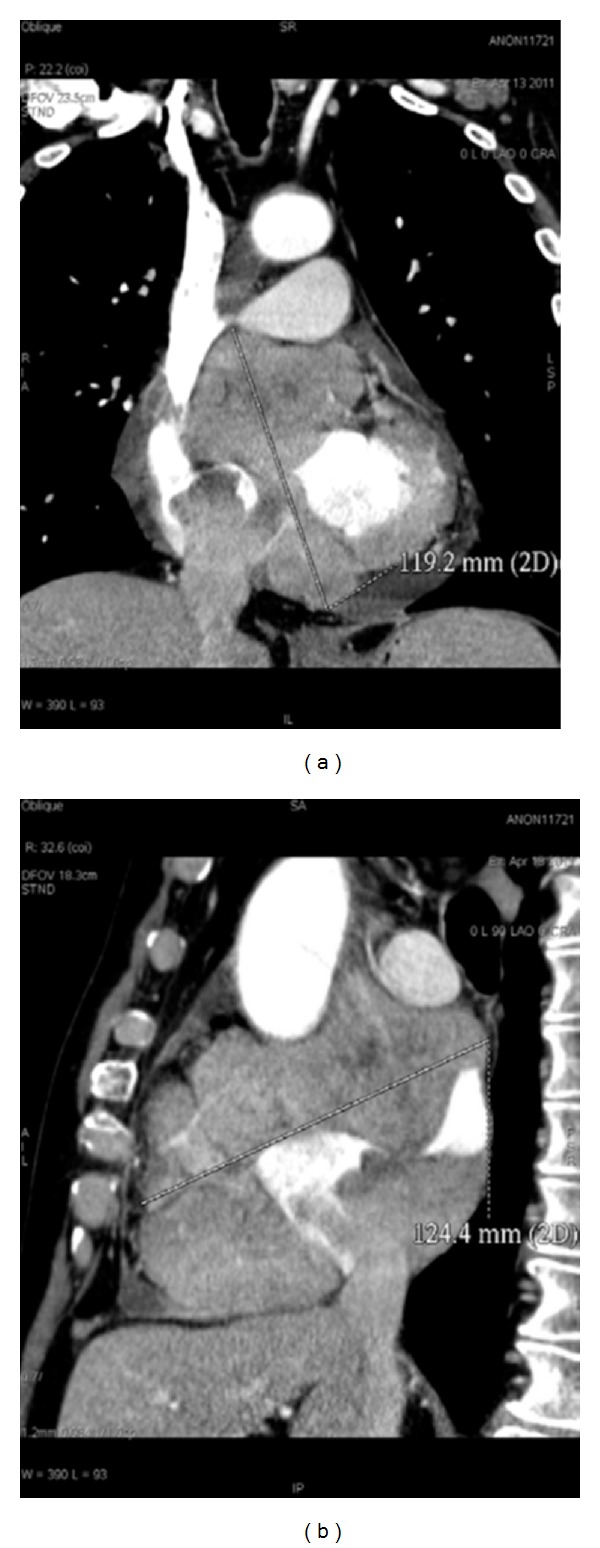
(a) Coronal reconstruction; (b) sagittal reconstruction. CT shows the presence of a 119.2 mm × 124.4 mm right atrium myocardial mass, involving the auricle and the atrial septum.
